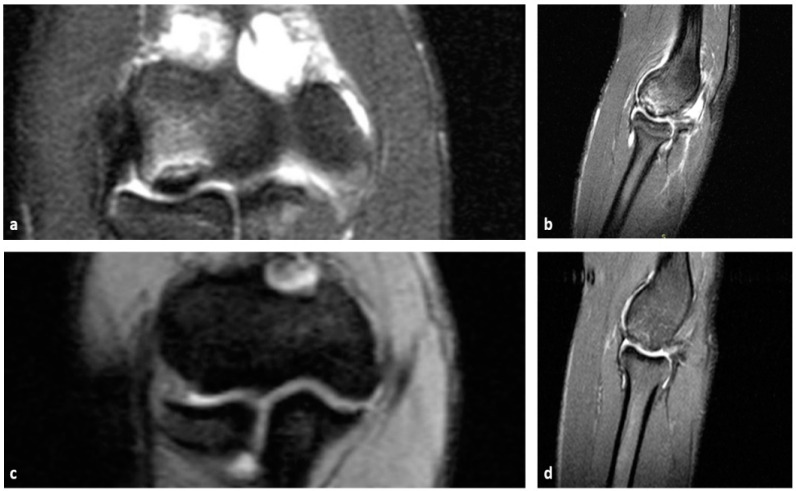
Preoperative coronal and sagittal PD fat-saturated MR images showing an unstable osteochondral defect (a,b). Postoperative coronal and sagittal PD fat-saturated MR images showing a satisfactory surgical outcome (c,d).
